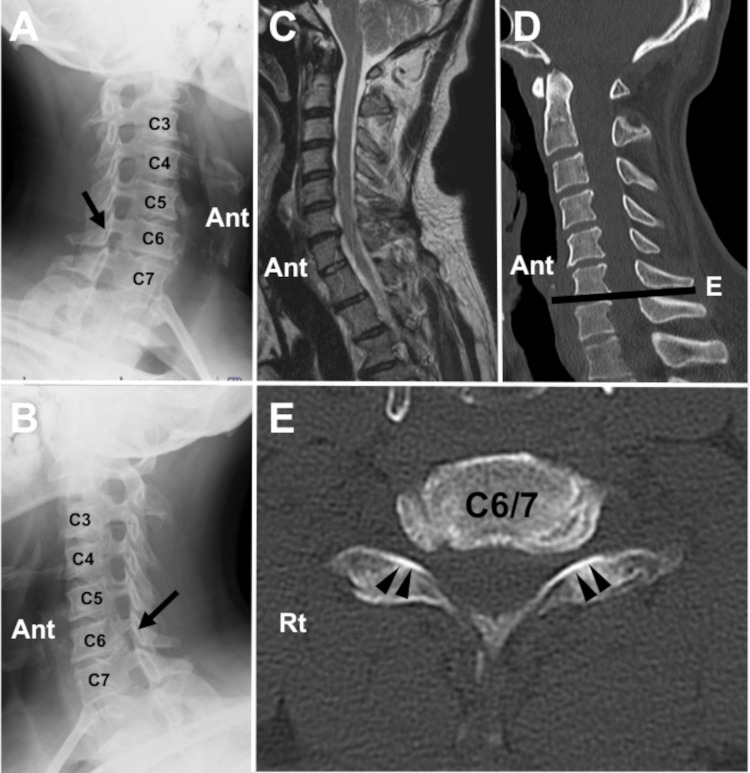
Preoperative radiographic images (Case 2). Oblique X-ray images reveal the stenosis in the bilateral C6/7 foramen (A, B, black arrows). A sagittal magnetic resonance image shows cervical spinal canal stenosis (C). (D-E) Computed tomography images disclose osteophytes forming the foraminal stenosis in the bilateral C6/7 foramen (black arrowheads) (Ant: anterior, Rt: right).
